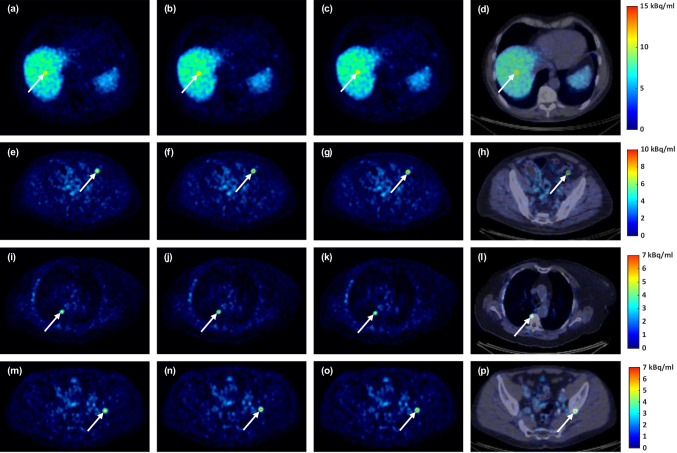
Image comparison of artificial soft tissue and bone lesions. From left to right, the columns show non-PRC, tissue-independent PRC, TDSV PRC, and fused PET/CT image for the TDSV PRC. Soft tissue_1_: (a)-(d). Soft tissue_2_: (e)-(h). Bone_1_: (i)-(l). Bone_2_: (m)-(p)
